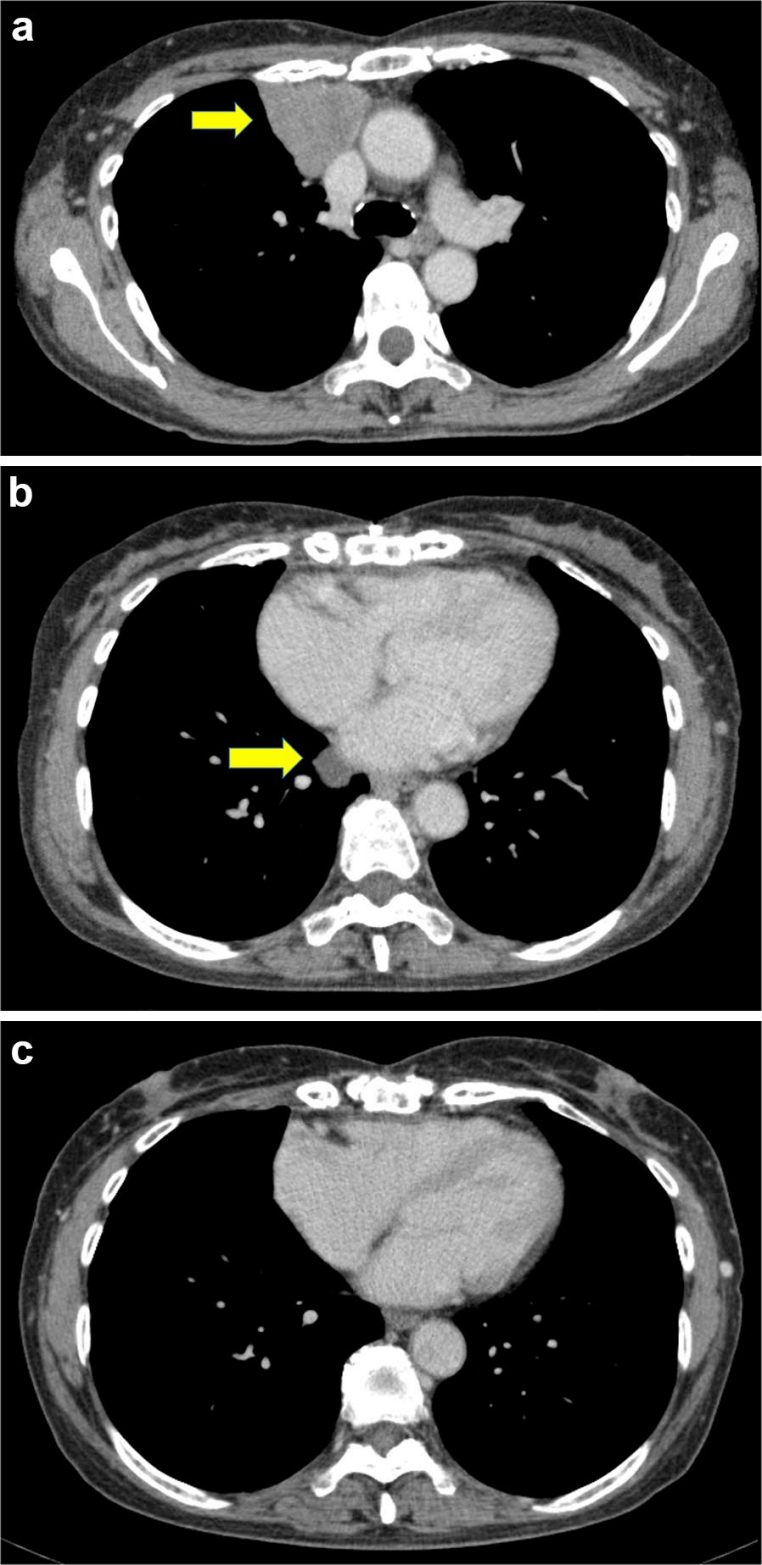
Computed tomography (CT) findings. a Local recurrence of thymoma at the first relapse. b A pleural dissemination of thymoma at the second relapse. c Complete remission following cyclosporine therapy
